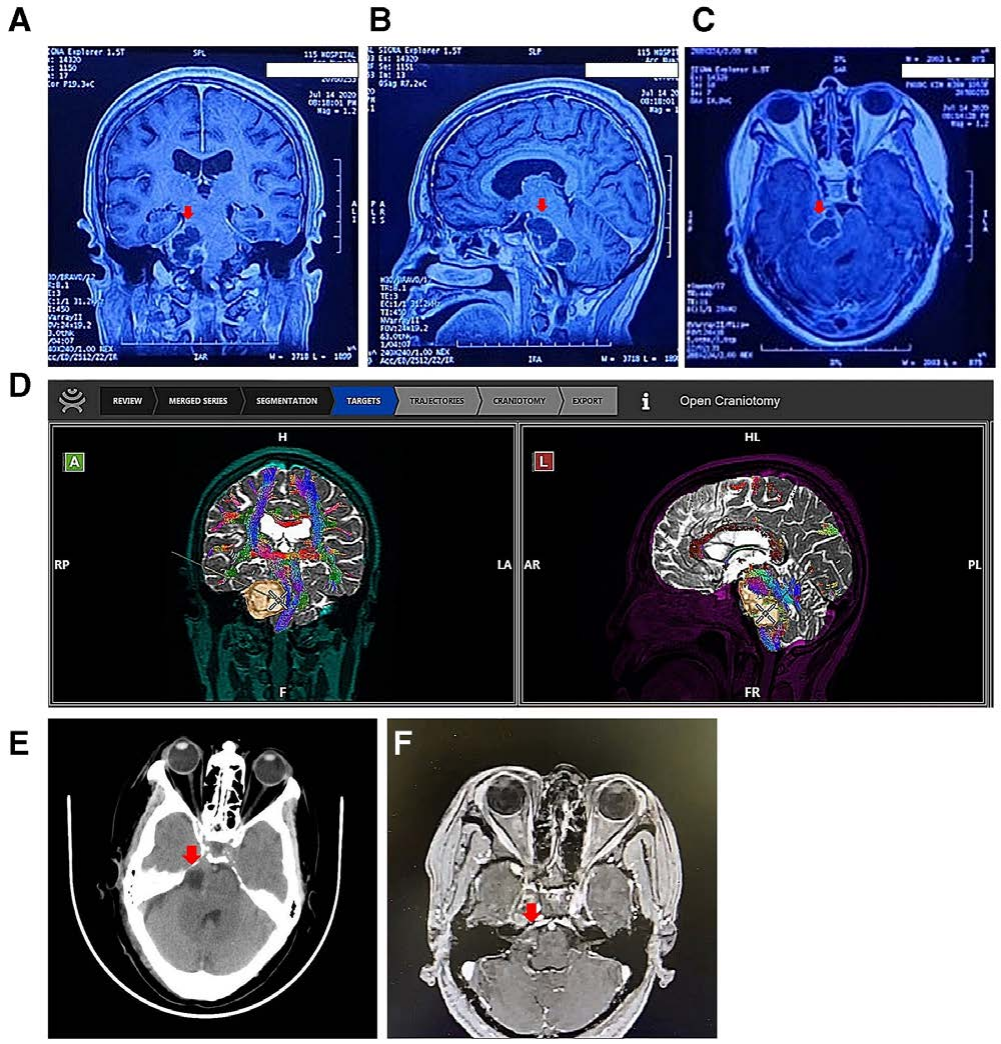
The tumor at CPA in MRI before the operation. A 4.8 × 1.8 × 2.6 cm uniform density tumor at the right CPA in T1-weighted MRI, in all 3 planes: (A) axial, (B) sagittal, and (C) coronal view, (D) and with DTI view of the tumor. Red arrow point at the tumor position. (E) The coronal view, 3 months after the operation. (F) The coronal view, 24 months after the operation. Red arrow points at the tumor position. CPA = cerebellopontine angle, DTI = diffusion tensor imaging, MRI = magnetic resonance imaging.
